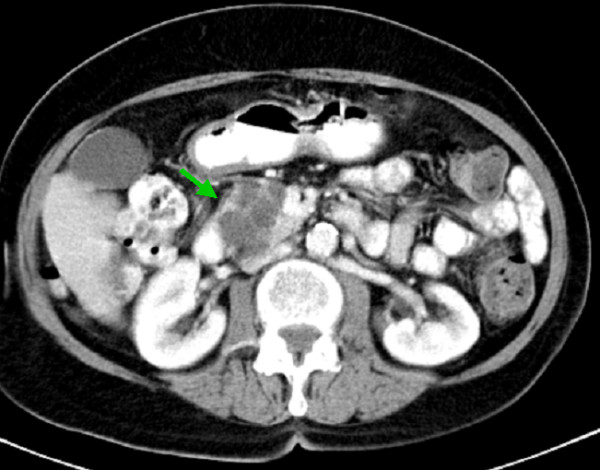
Benign multicystic mesothelioma of the head of the pancreas: computed tomography scan of the abdomen shows a multicystic lesion of the head of the pancreas with dilated pancreatic duct and regional lymphadenopathy.
